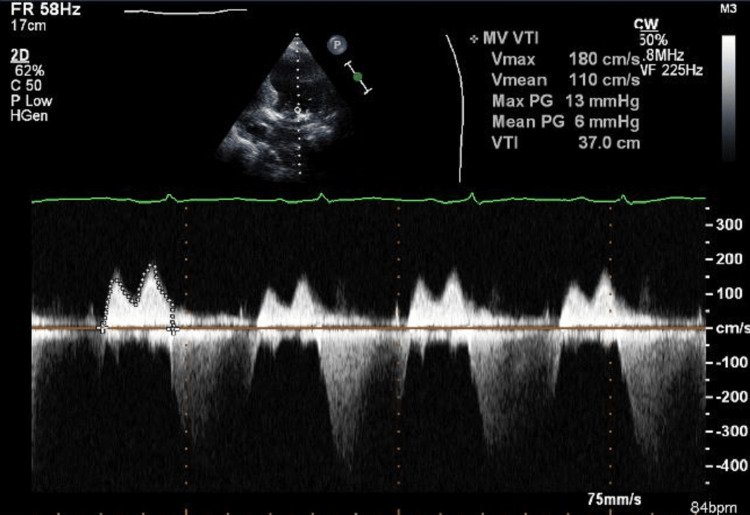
2D ECHO Doppler image showing features suggestive of MS 2D ECHO: two-dimensional echocardiography; MS: mitral stenosis.
